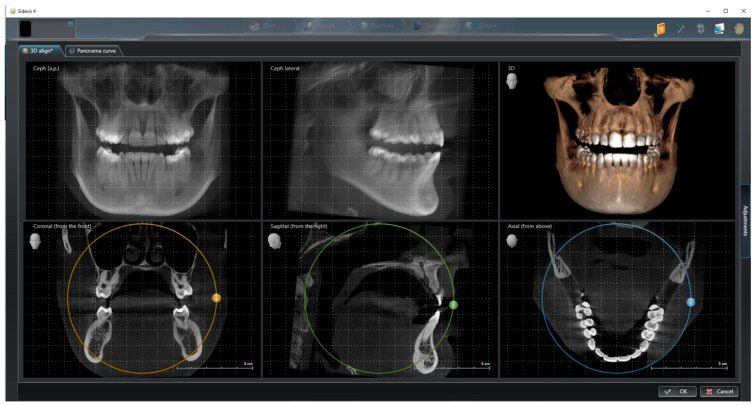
CBCT alignment to correct patient position by adjusting the tilt and rotation.
